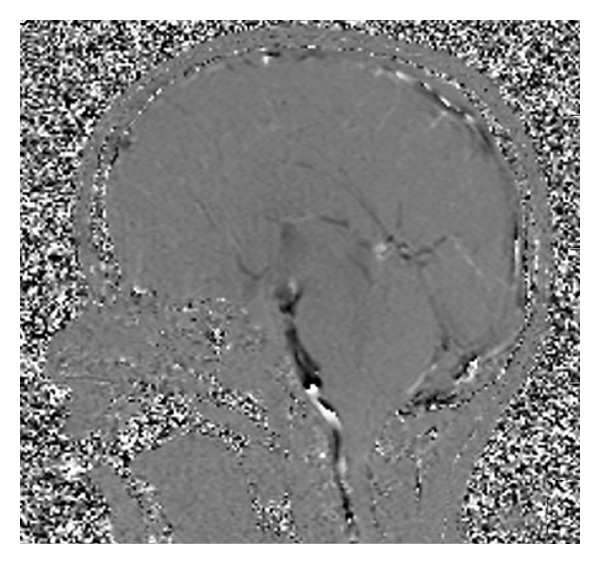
Postoperative MRI 2D phase contrast with third ventricle CSF flow evidence.
